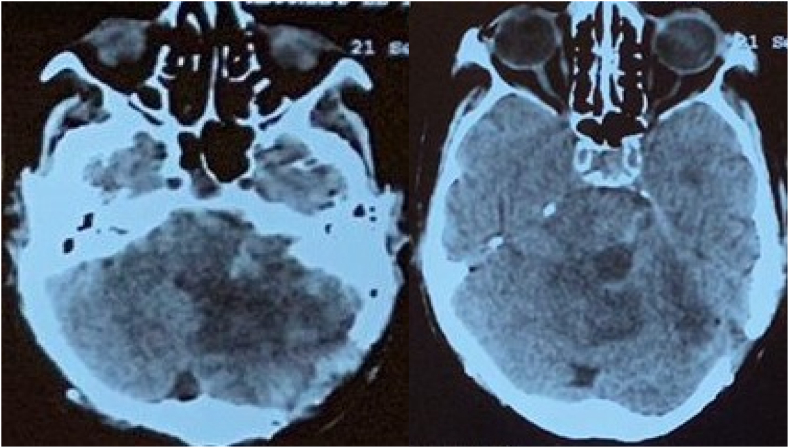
Immediate postoperative CT scan showing oedemato-hemorrhagic remodeling at the surgical site.
